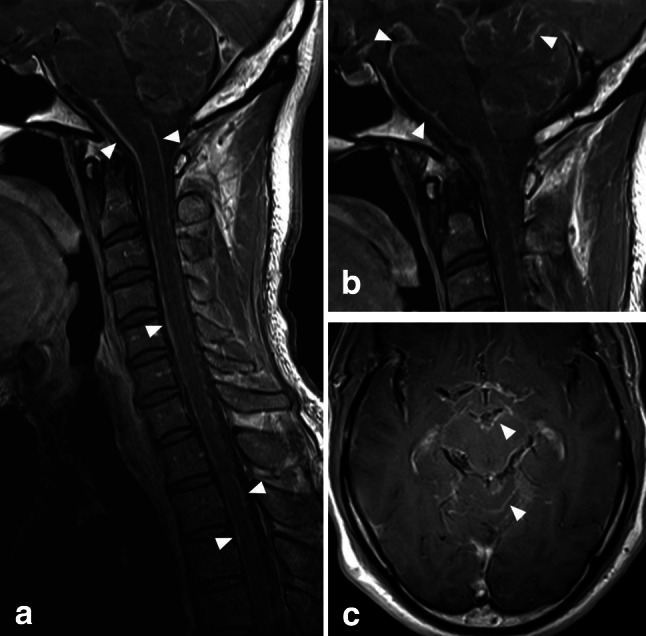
Post-gadolinium T1-weighted MR images of the cervical spine (a, b) and brain (c), demonstrating diffuse, mildly nodular leptomeningeal enhancement over the surface of the spinal cord, brain stem and cerebellum (*arrowheads*)
